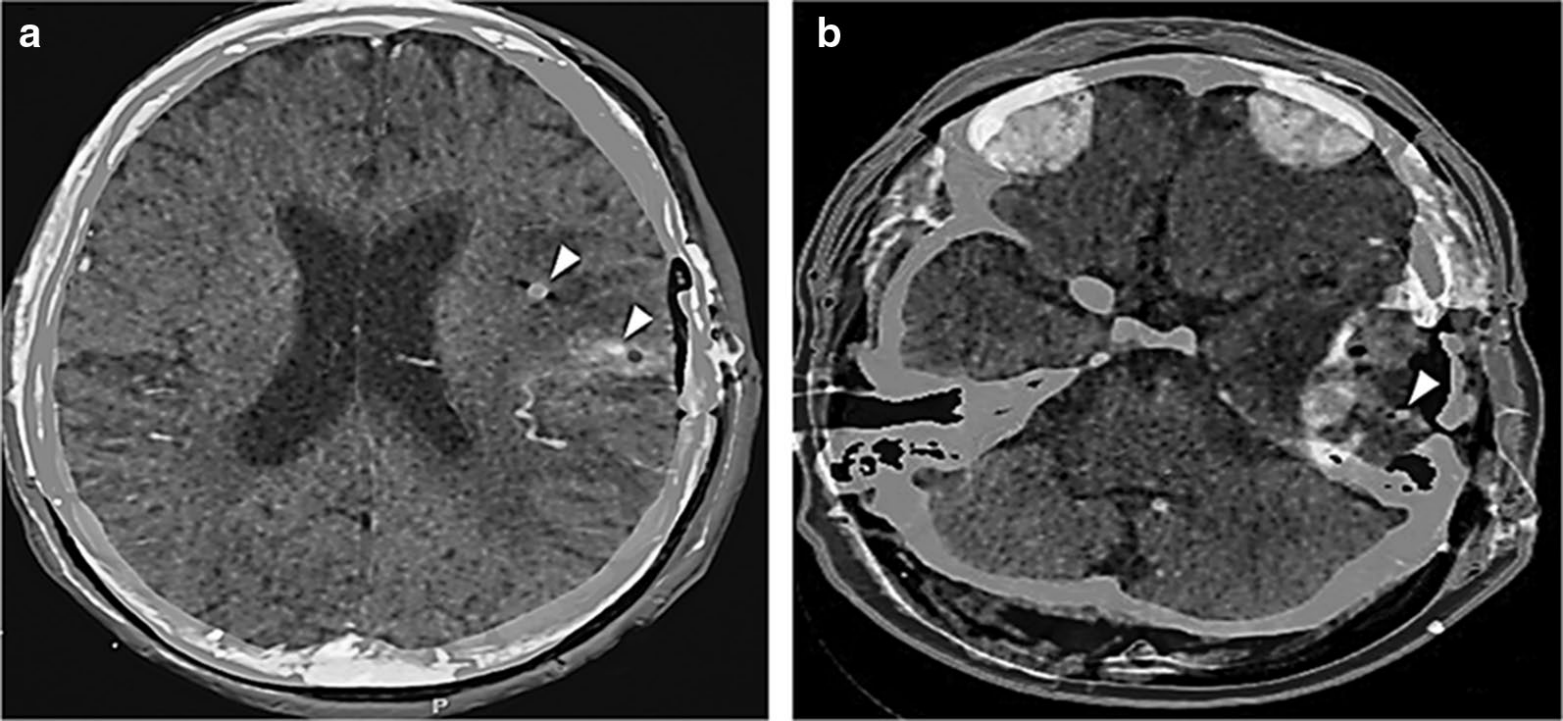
Catheter placement imaging. Brain CT and MRI superimposed delineating catheter placement and tumor margins. Patient 1 had one catheter tip placed in non-contrast enhancing area and the second in contrast enhancing tissue (a). Patient 2 had one catheter placed in non-contrast enhancing tissue (b). Superimposed images denote contrast enhancement in green. The white triangle indicates placement of catheter tips
